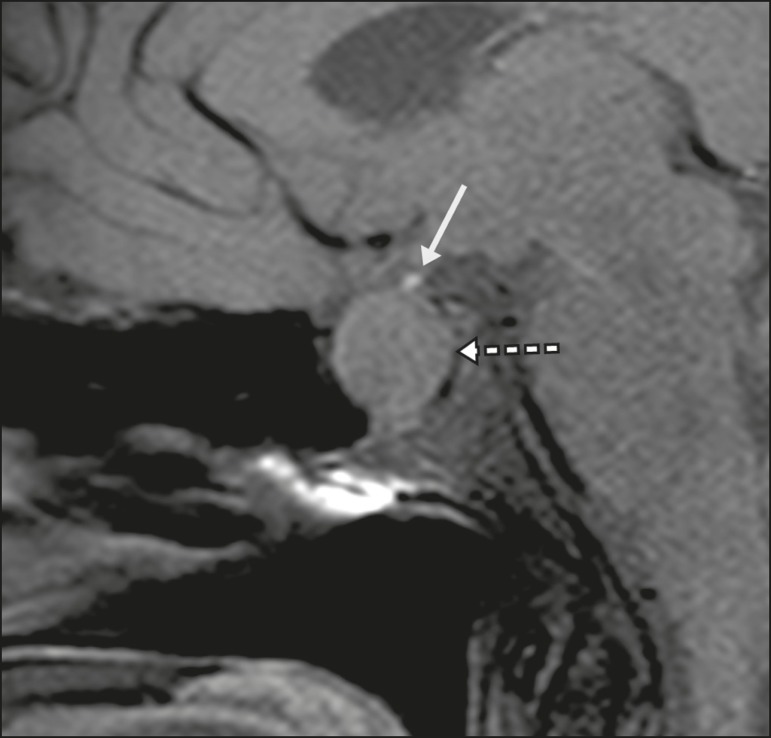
Sagittal T1-weighted MRI sequence with fat saturation, showing an ectopic
